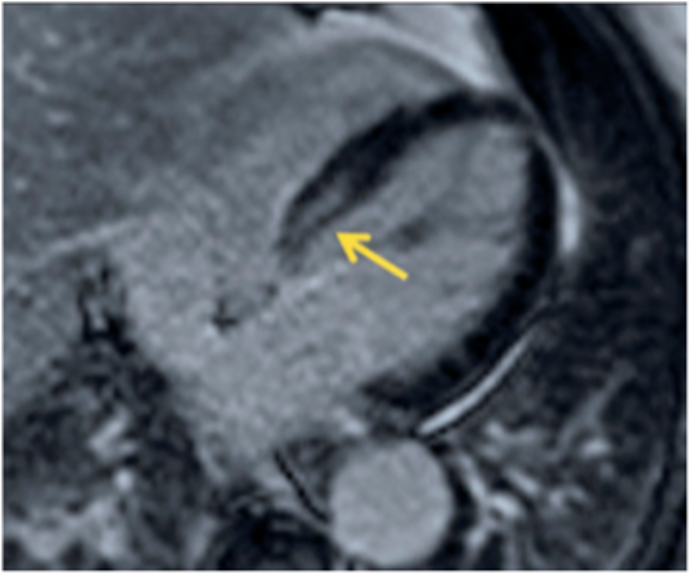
Cardiac MRI showing evidence of myocarditis.
